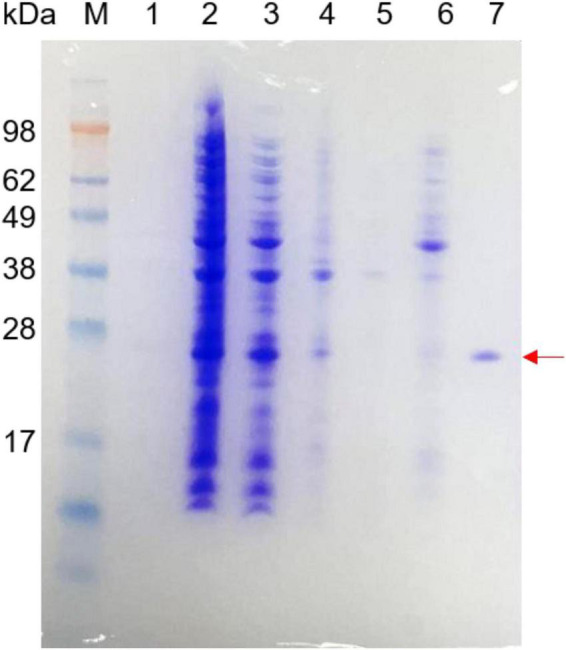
SDS-PAGE of purified recombinant Bgl-16A protein. Line 1: supernatant of fermentation liquid; Line 2: all cells after ultrasonically broken; Line 3: supernatant of cells after ultrasonic fragmentation; Line 4: precipitate of cells after ultrasonic fragmentation; Line 5: flow liquid through the Ni-NTA column; Line 6: 40 mM imidazole eluent; Line 7: purified enzyme obtained by 200 mM imidazole eluent liquid.
